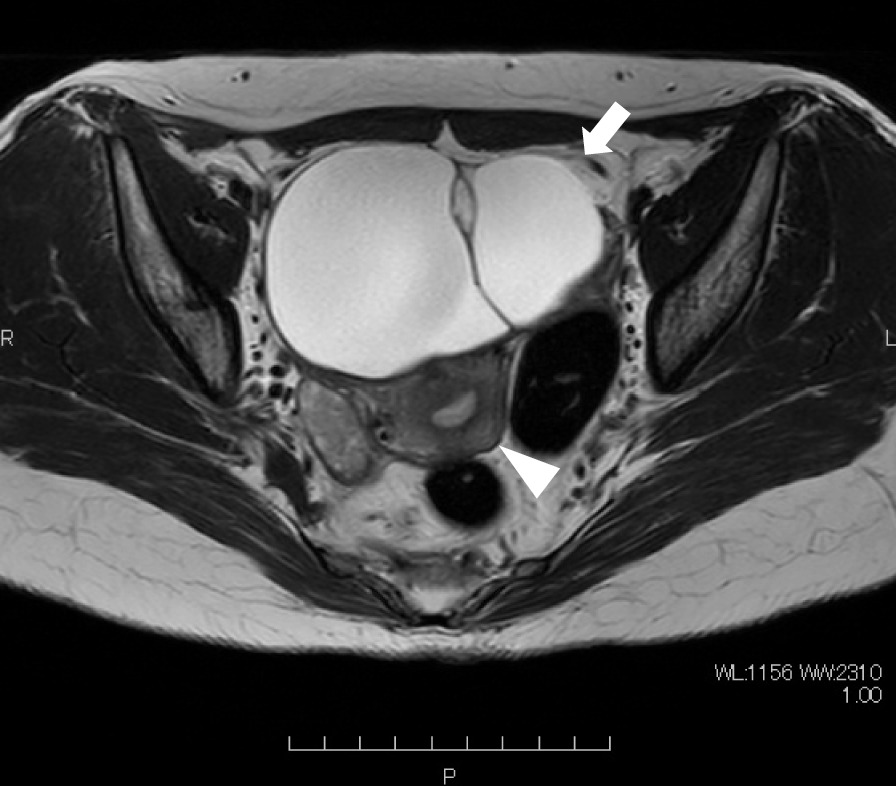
Axial T2-weighted magnetic resonance image of the adnexal mass with multiple cystic cavities in the left adnexal region (arrow). The mass was identified as adjacent to the uterus with the endometrium (arrowhead)
